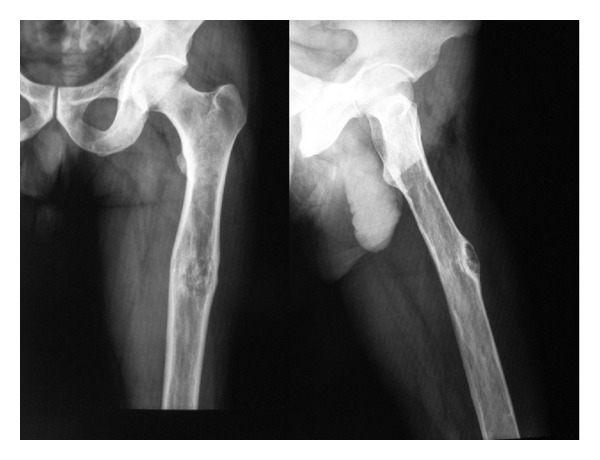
Plain radiographs of the left femur demonstrating moderate expansion in bone, including lytic areas with a cortical defect at the anterolateral aspect of the upper third of the left femoral shaft and cortical thinning.
